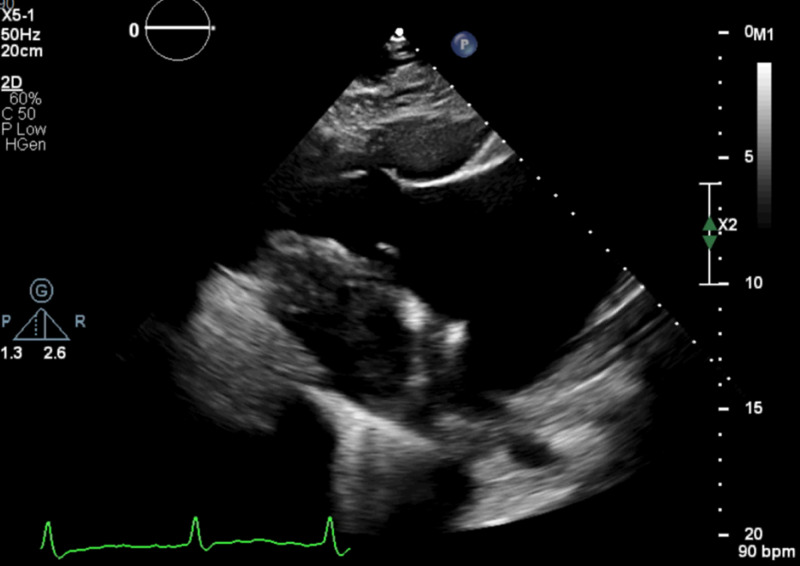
Echocardiographic image with wide open tricuspid valve with minimal visualization of leaflets
